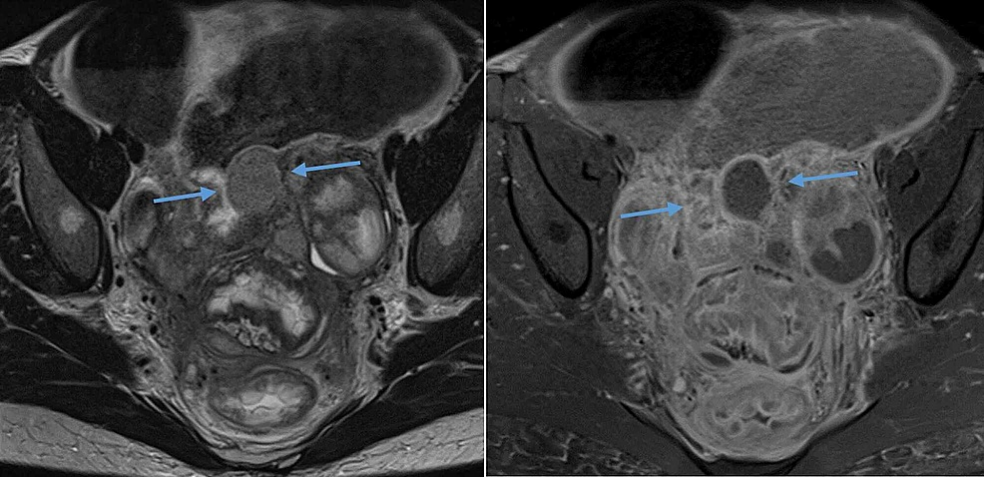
Axial T2 weighted and contrast-enhanced T1 weighted MRI pelvis showing the multiple pelvic abscesses and left-sided tubo-ovarian abscess. Note the position of one of the abscesses adjacent to the transition point in the colon (blue arrows). The distal sigmoid colon loops are thickened and inflamed, while the proximal loops are distended.
